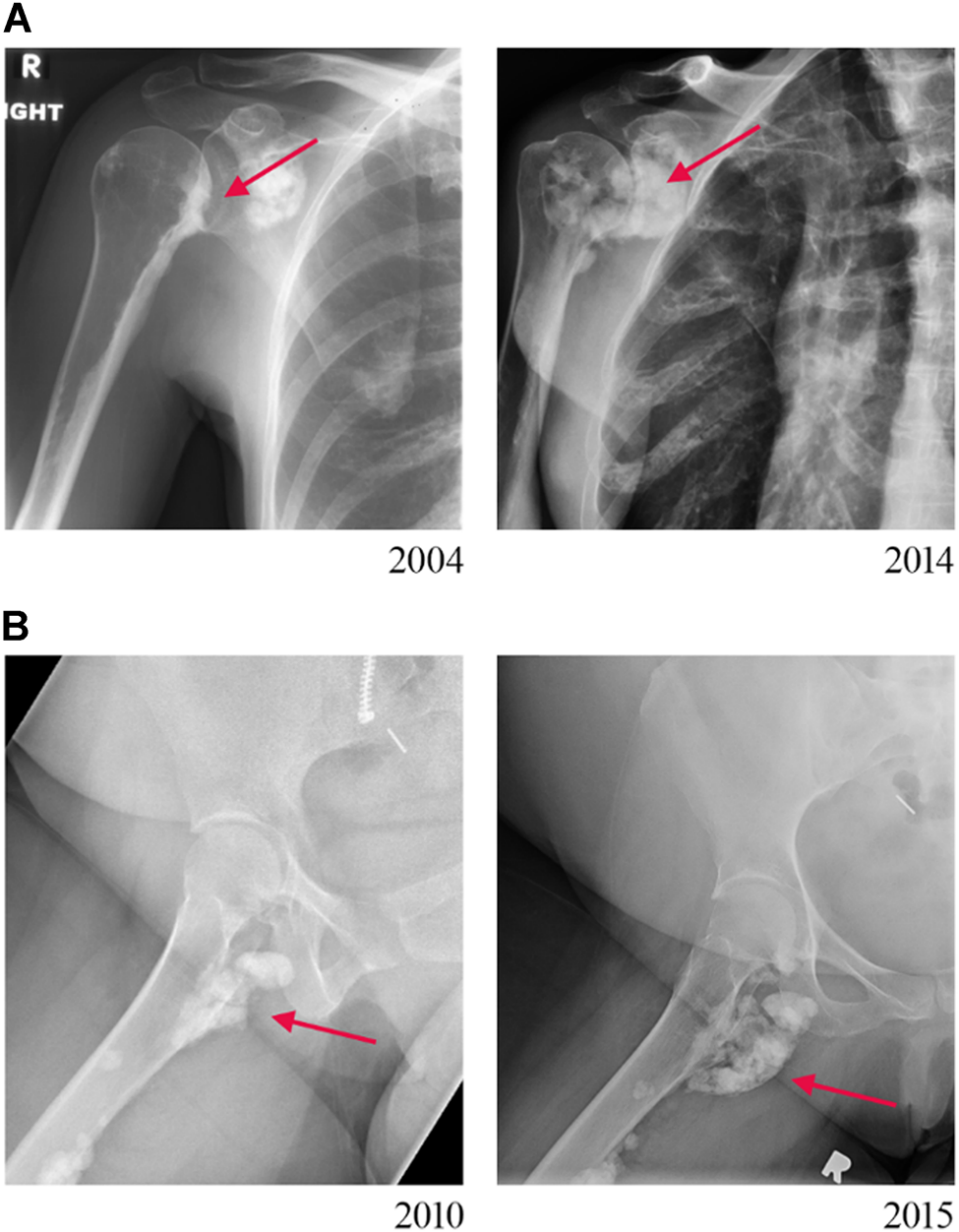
Radiographic progression in melorheostosis. (*A*) Comparison of radiographs from 2004 (left) and 2014 (right) showing progression of both skeletal and extraosseous lesion of melorheostosis (Melo‐21; *MAP2K1* mutation negative). (*B*) Comparison of radiographs from 2010 (left) and 2015 (right) showing evidence of disease progression in the extraosseous lesion around patient's right hip joint (Melo‐6; MAP2K1‐mutation positive).
